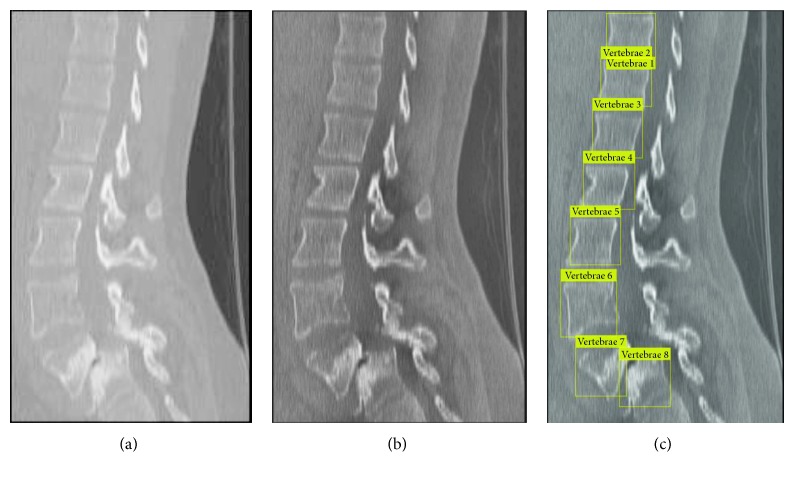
Another detected result: (a) original CT image; (b) the enhanced image by the CLAHE method; (c) the final detected result.
